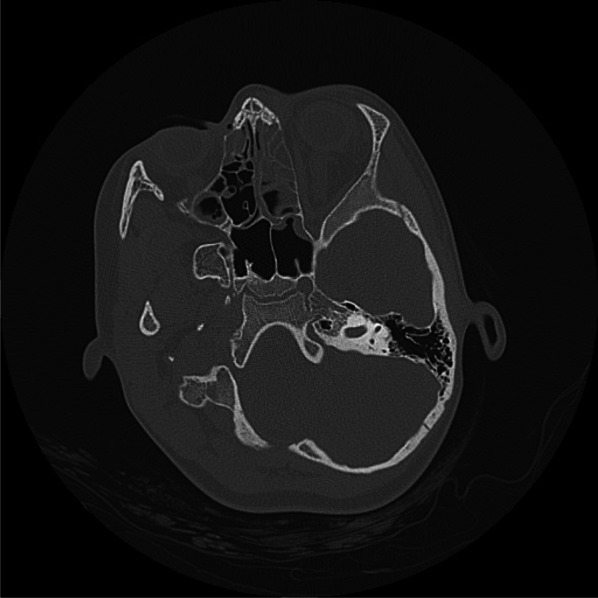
Patients CT scan showing opacification of the left frontal, ethmoidal and both maxillary sinus
